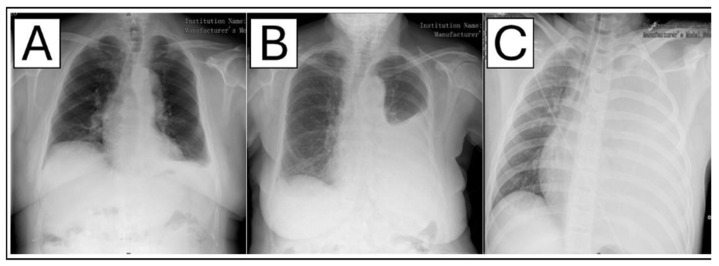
Semi-quantitative classification of left-sided hemothorax on chest radiography at admission. Representative chest X-rays showing (A) small left-sided hemothorax, defined as blunting of the left costophrenic angle; (B) moderate left-sided hemothorax, extending to approximately half of the left hemithorax and partially obscuring the cardiac silhouette; and (C) massive left-sided hemothorax, with near-complete opacification of the left hemithorax.
